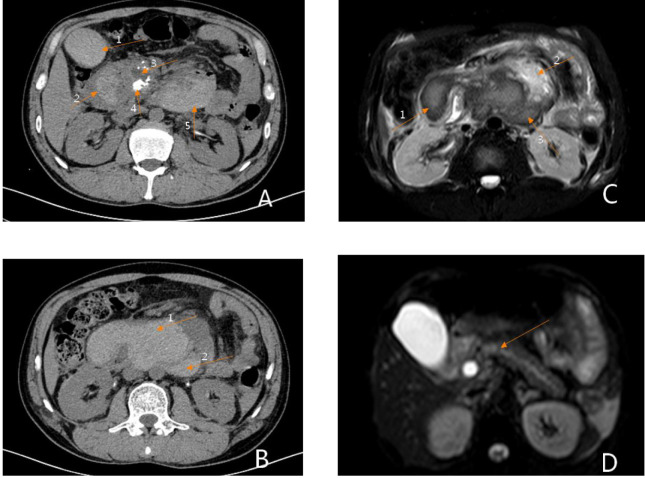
Preoperative imaging findings. (A) Phase III dynamic enhanced abdominal CT: Arrow 1 shows an intragallbladder hematoma, arrow 2 shows an intraduodenal hematoma, arrow 3 shows dilation of the main pancreatic duct, arrow 4 shows uneven enhancement of the head of the pancreas, suggestive of pancreatitis, and arrow 5 shows a retroperitoneal hematoma. (B) Phase III dynamic contrast-enhanced abdominal CT: Arrow 1 shows an intraintestinal hematoma, arrow 2 shows a retroperitoneal hematoma. (C) Abdominal MRI: Arrow 1 shows an intraduodenal hematoma, arrow 2 shows normal pancreatic tissue, and arrow 3 shows a retroperitoneal hematoma. (D) Abdominal MRI: This image shows a fully distended pancreatic duct.
